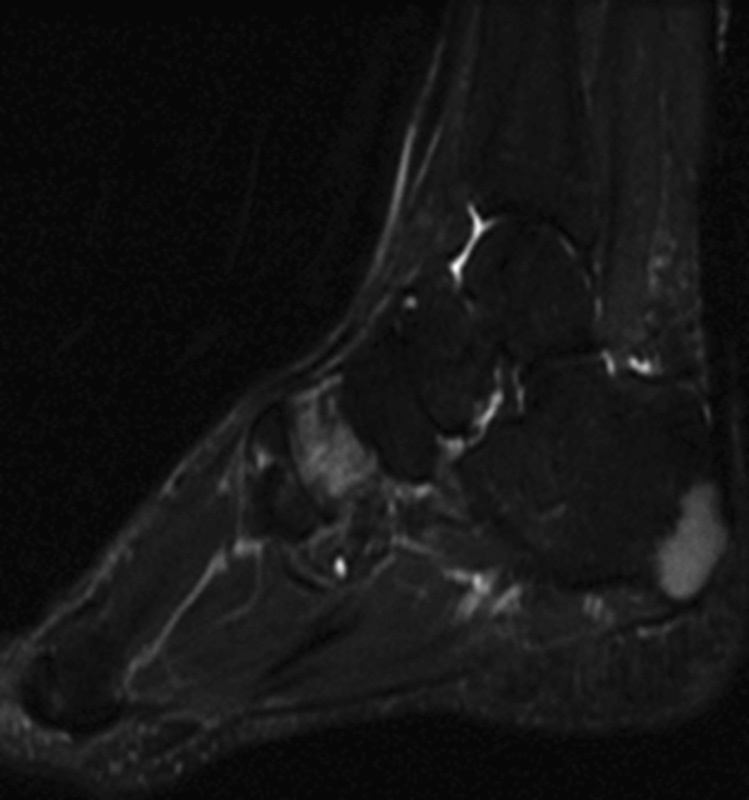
Hyperintense lesions in calcaneus and cuneiform (T2-weighted magnetic resonance imaging section).
